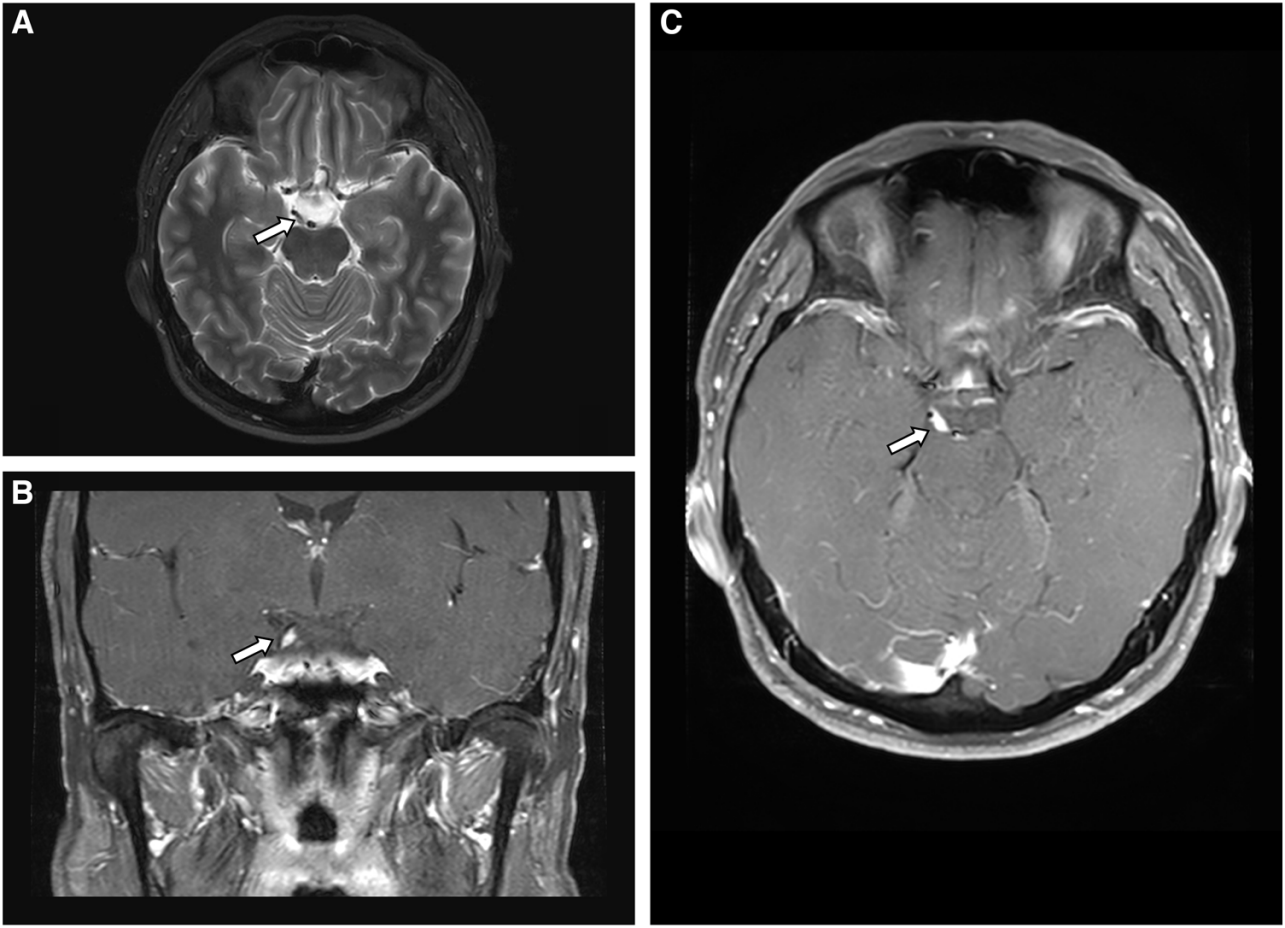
Axial T2-weighted (A) as well as coronal (B) and axial (C) T1-weighted MRI following administration of gadolinium showing thickening and enhancement of the right oculomotor nerve in its interpeduncular and suprasellar cisternal course.
